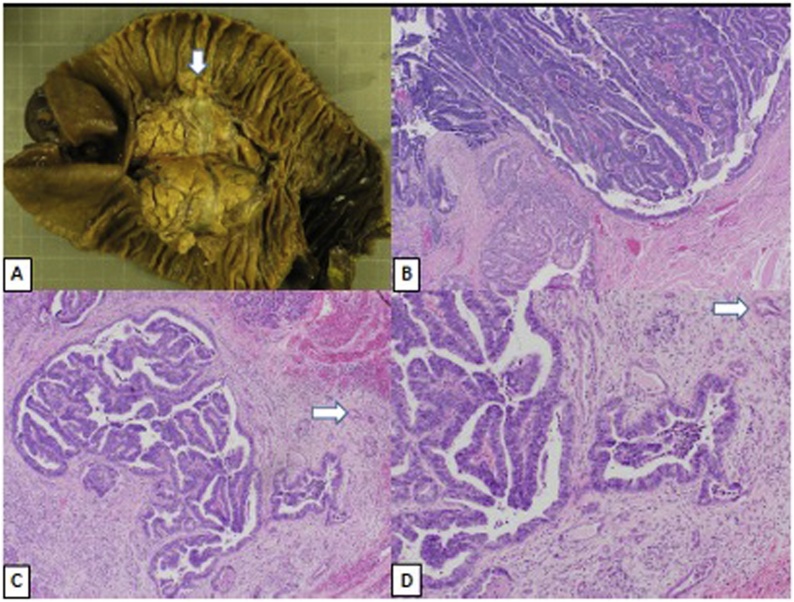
(A) Coronal T2 weighted MR abdomen examination showing a pancreatic head ductal lesion (red arrow). (B) Axial T2 weighted MR and (C) axial contrast enhanced CT abdomen showing normal pancreatic body and tail.
